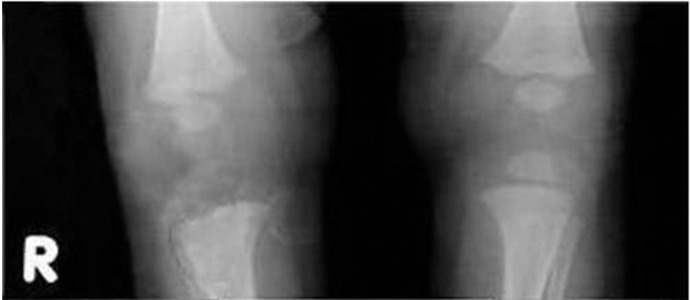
X-ray of the lower limbs 15 days after admission (1 month of age) shows osteolysis of the upper metaphysis of the right tibia.
